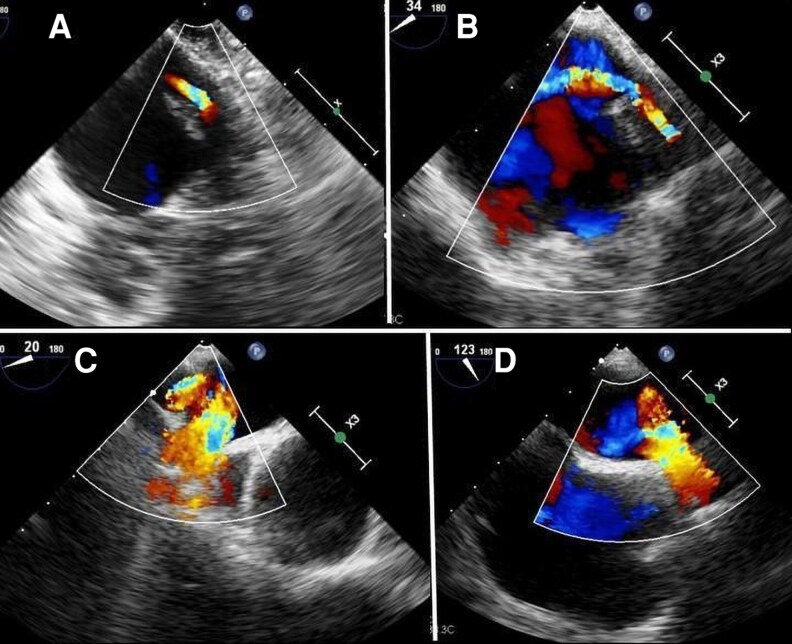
TOE is useful for identifying and confirming PV stenosis and for estimating pressure gradients. Colour Doppler with a low-velocity scale (< 40 cm/sec) enhances PV visualization: turbulent flow across the stenotic tract generates a mosaic pattern due to acceleration and aliasing. (*A*): 2D-colour-Doppler of the LUPV (from LAA view) showing aliasing consistent with stenosis. (*B*): 2D-colour-Doppler of the LUPV demonstrating multicolour aliasing due to increased flow velocity from multiple stenosis. (*C*): 2D-colour-Doppler of the RLPV (from a superior ME short-axis view) showing turbulent flow (*D*): 2D-colour-Doppler of the RUPV (from the ME modified bicaval view) with aliasing related to stenosis. LAA: Left atrial appendage; LUPV: Left upper pulmonary vein; ME: Mid-oesophageal; PV: Pulmonary vein; RLPV: Right lower pulmonary vein; RUPV: Right upper pulmonary vein; TOE: transoesophageal echocardiography.
